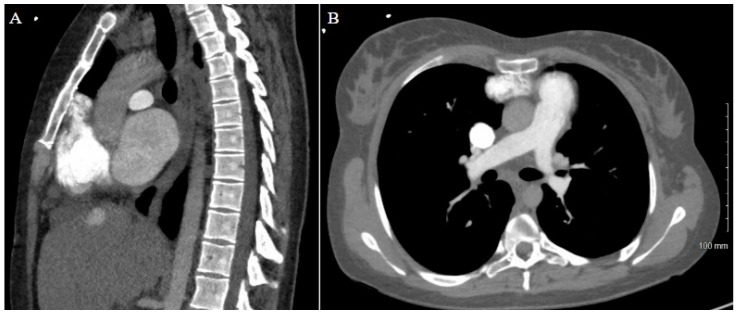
Patient 2 CT chest w/contrast. (A) Sagittal view and (B) axial view reveal extensive adhesions between right atrium and inferior retrosternum.
